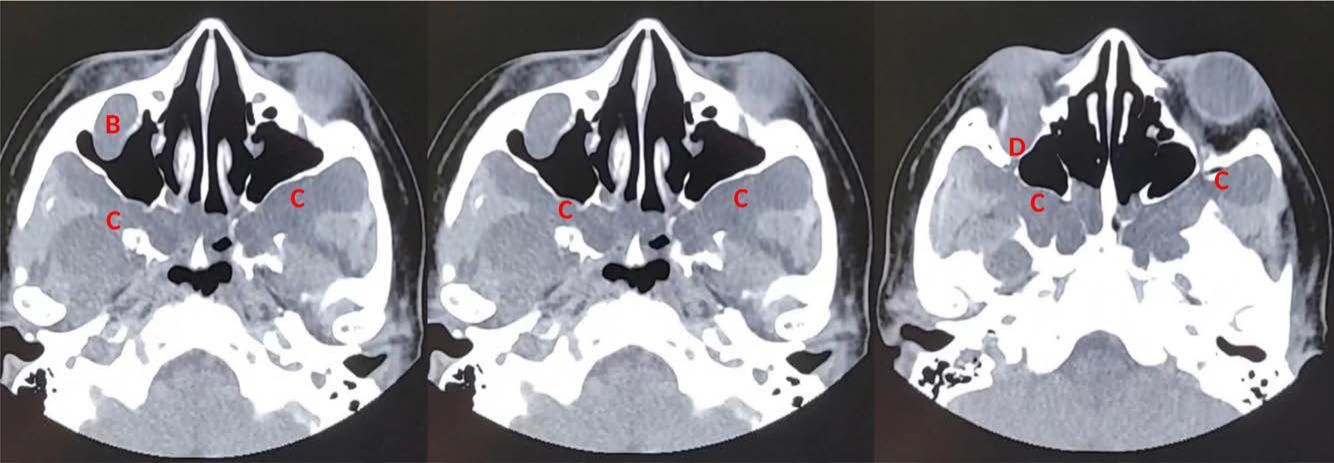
The axial images of the orbital CT reveal lesions characterized by low-density shadows (B), extensively involving the right infraorbital canal, bilateral nasopharynx, infratemporal fossa, pterygopalatine fossa (C), and the muscle spaces in the middle cranial fossa. Additionally, there is enlargement of the right infraorbital canal (D). CT = computed tomography.
